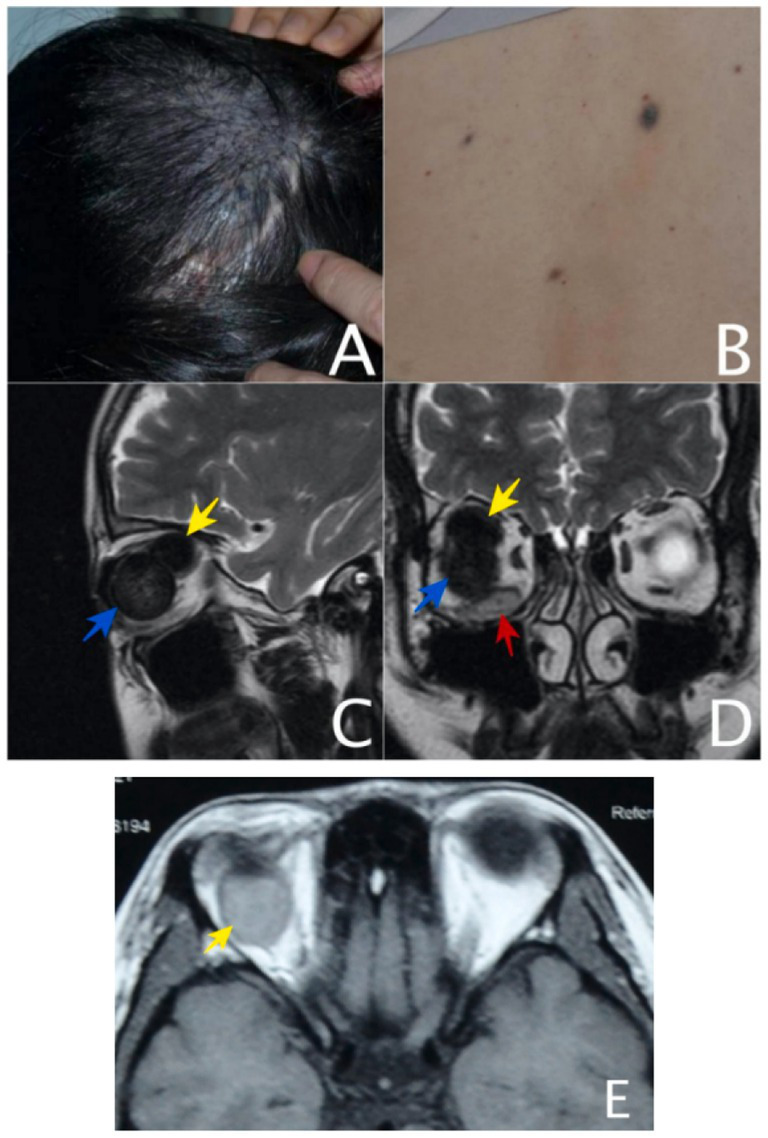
(A) A large blue nevus on the right upper scalp. (B) Small blue nevi on waist skin. (C) MR images on T2WI: Sagittal scan of the right orbit: showed an oval-shaped, homogeneous hypointense mass (the yellow arrow) relative to extraocular muscle above and behind the orbital implant (the blue arrow), the right inferior rectus muscle significantly thickened and slight hyperintense signal. (D) MR images on T2WI: coronal scan: showed an oval-shaped, homogeneous hypointense mass (the yellow arrow) relative to extraocular muscle above and behind the orbital implant (the blue arrow), the right inferior rectus muscle (the red arrow) significantly thickened and slight hyperintense signal. (E) MR images on T1WI: horizontal scan: showed an oval-shaped, homogeneous slight hyperintense mass (the yellow arrow) relative to extraocular muscle and behind the orbital implant (the blue arrow).
